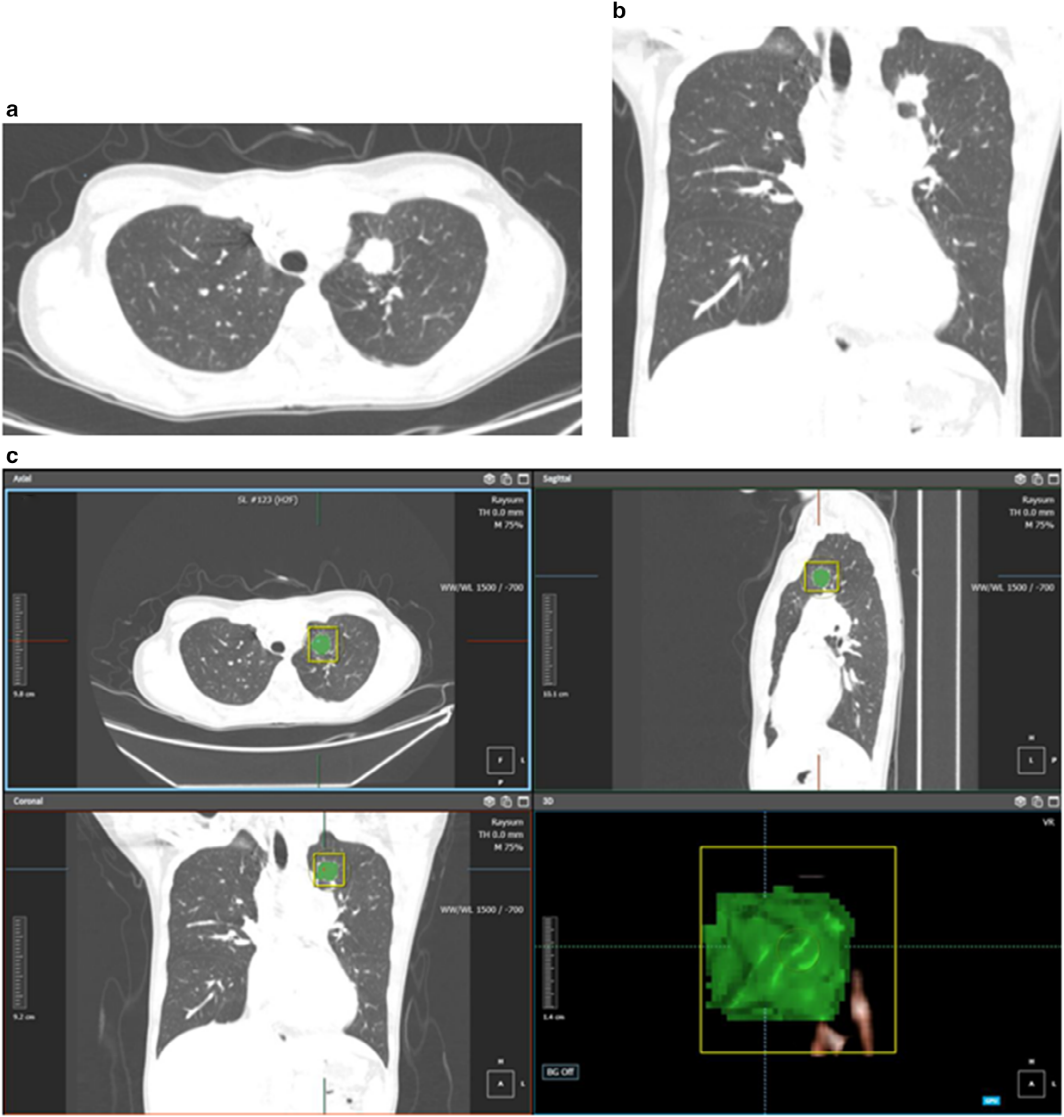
CT images and radiomic analysis of a 34‐year‐old female with adenocarcinoma positive for PD‐L1 expression. Axial (a) and coronal (b) images of the initial contrast‐enhanced CT scan show a 2.3 cm nodule in the left upper lobe which appears as a pure solid nodule with spiculation and pleural attachment. Multiple metastatic left hilar, bilateral mediastinal and left supraclavicular lymph nodes are present. The patient was diagnosed as having adenocarcinoma with high PD‐L1 expression (tumor proportion score = 80%) and epidermal growth factor receptor wild‐type via ultrasound‐guided neck lymph node biopsy. (c) Radiomic feature extraction was performed with segmentation of the volume of interest of the left upper lobe nodule. The Rad‐score is 1.350, which is higher than the cutoff value of −0.715 for positive PD‐L1 expression. CT, computed tomography; PD‐L1, programmed death ligand 1.
